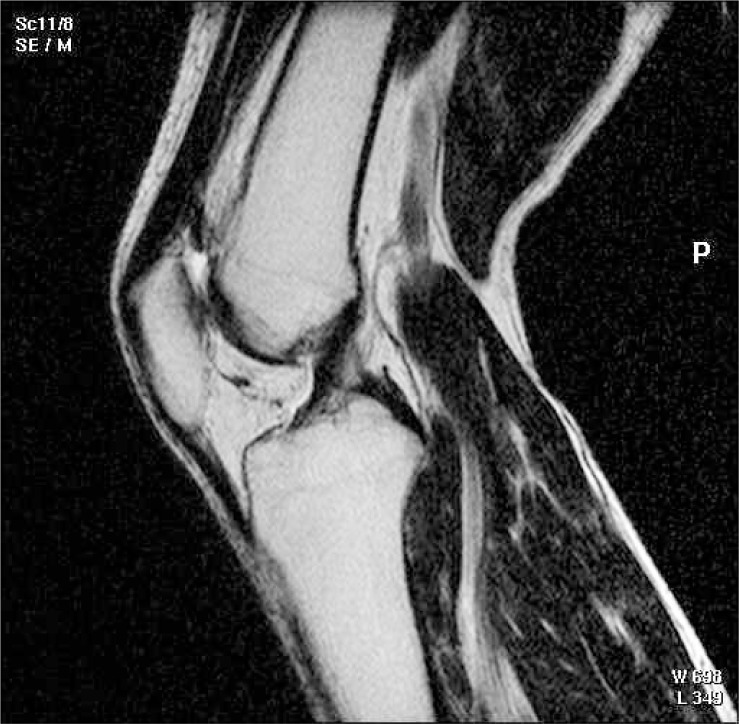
Anterior cruciate ligament (ACL) flexion MRI technique.
